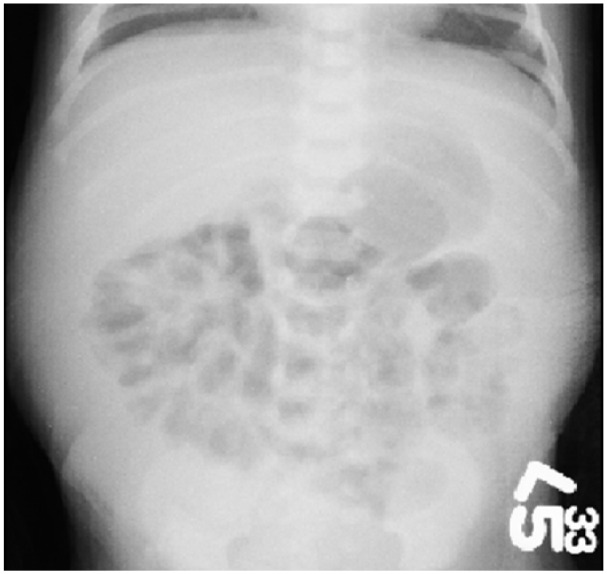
Abdominal radiograph, anterior-posterior view of infant in Case 2. The intestinal gas pattern is normal; however, the loops are centralized, a sign of free fluid in the peritoneal cavity.
